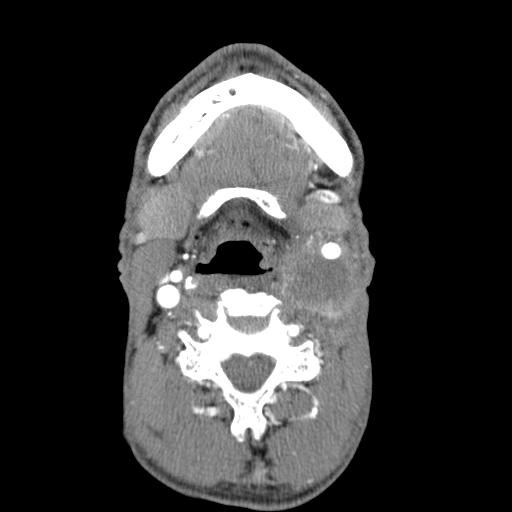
Cervical CT-image (axial view, at the hyoid bone level) showing adenopathy invasion into the left common carotid artery.
